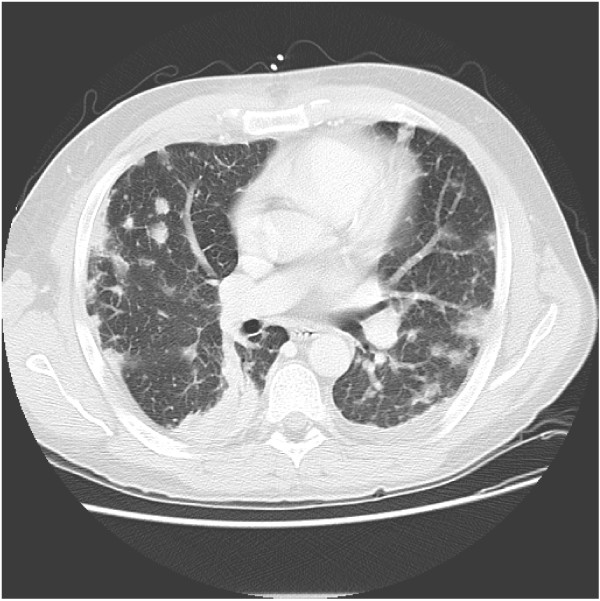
High-resolution (HR-)CT-scan at admission showing signs of severe pulmonary infiltration.
